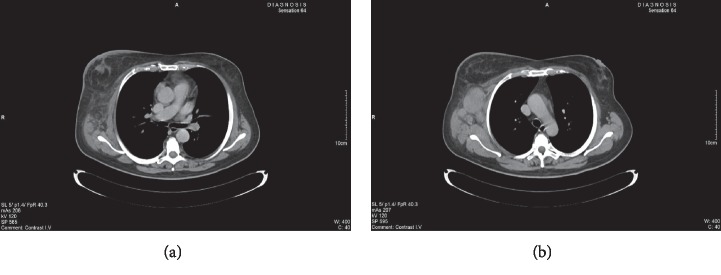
CT revealing breast lesion with partially ill defined borders, thickening of the breast skin, and lymphedema (a) and enlarged axillary lymph nodes (b).
